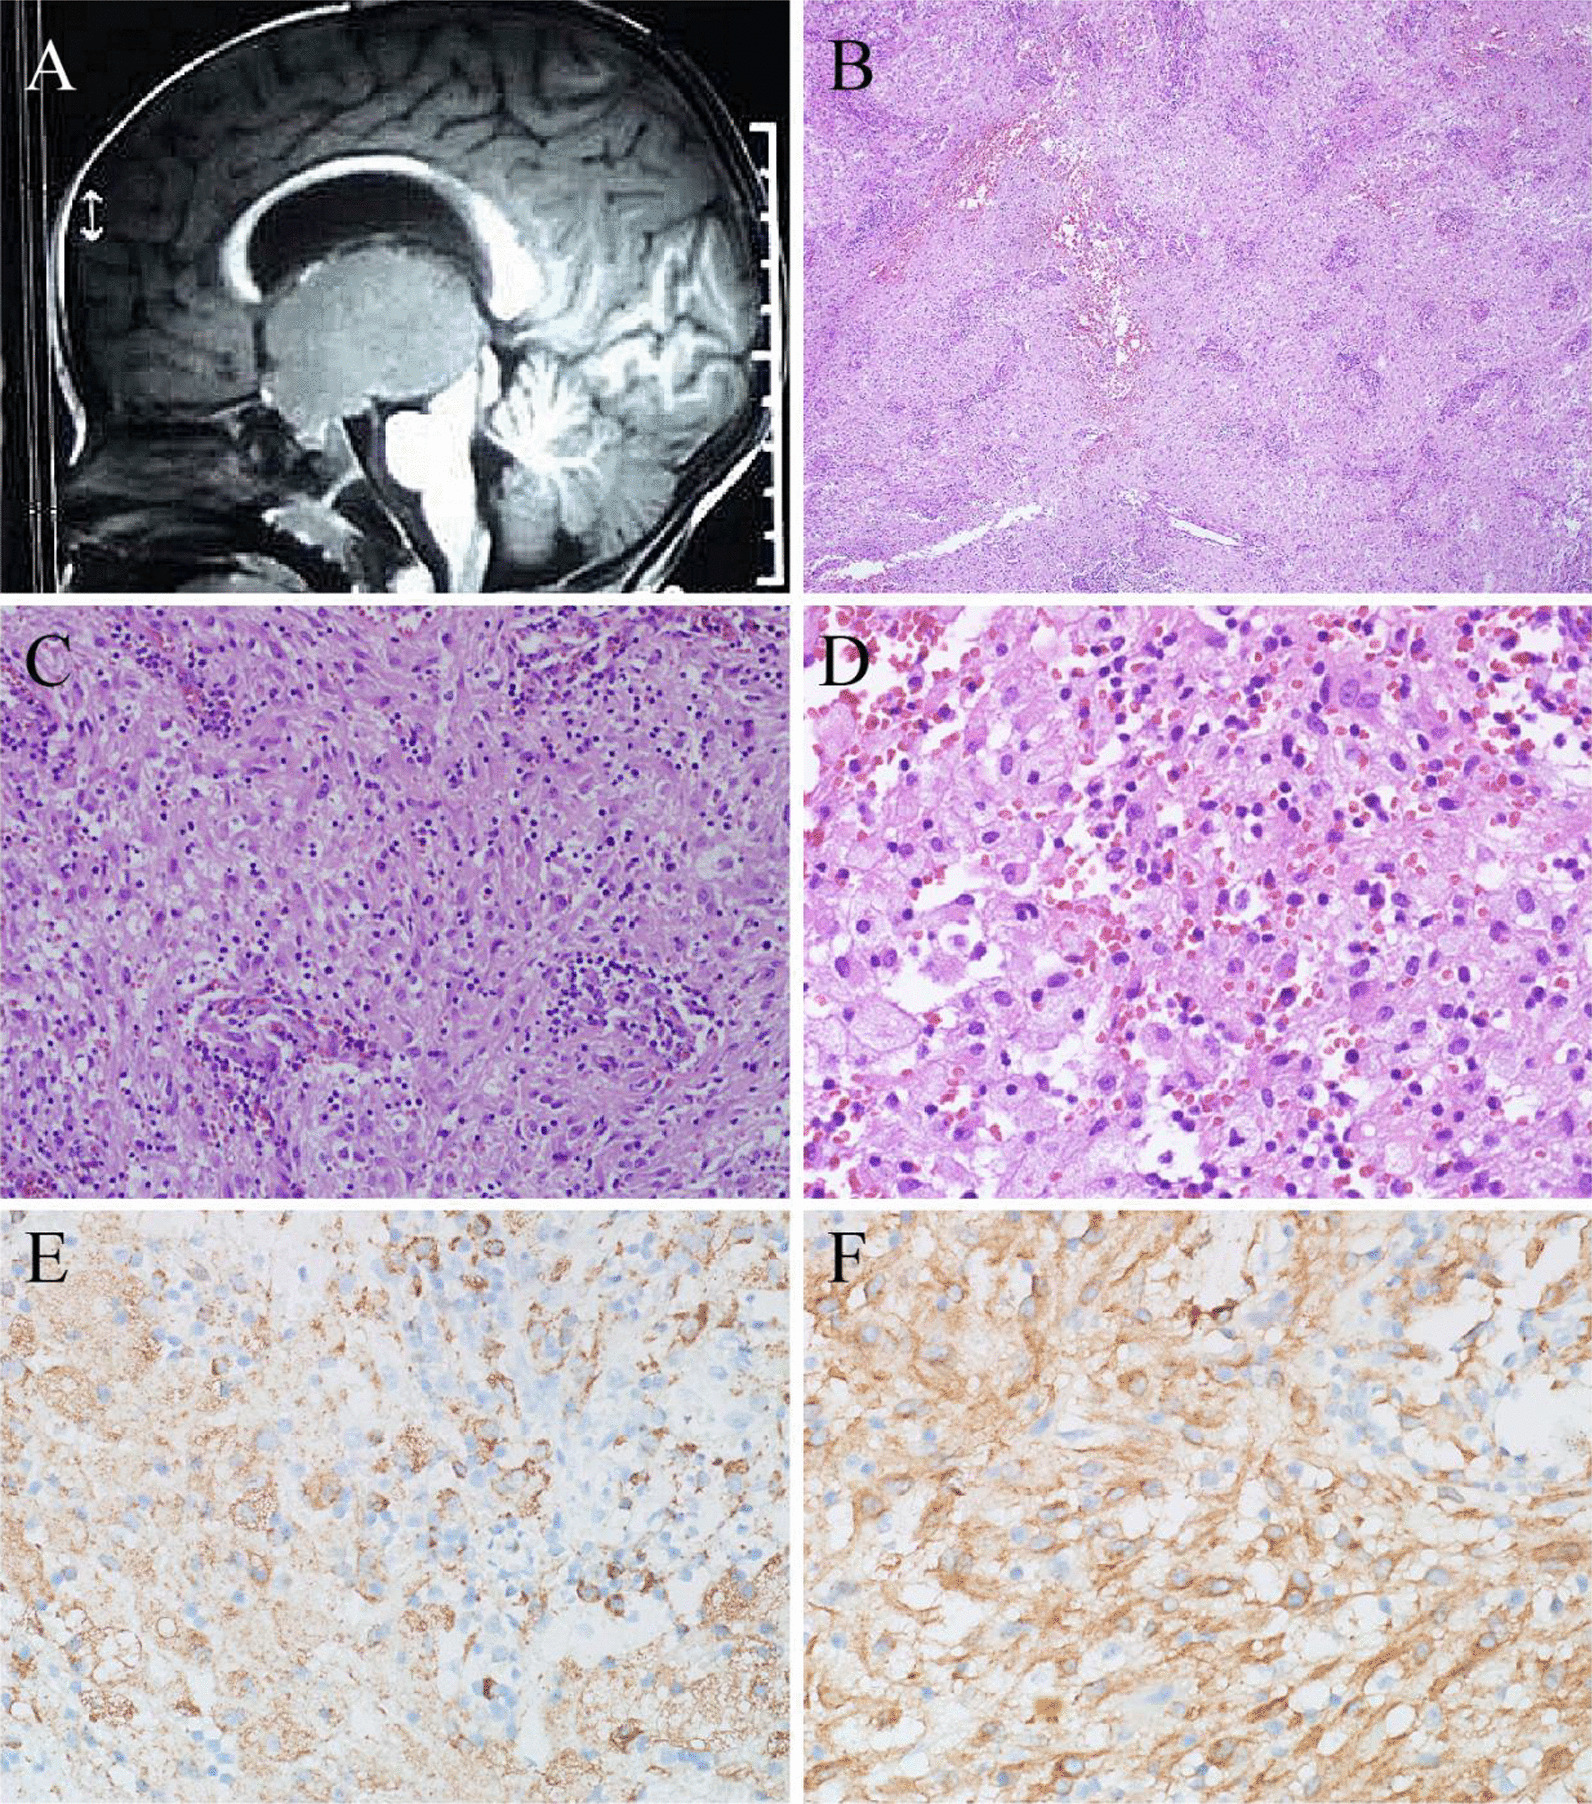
Radiological and pathological features of case 4. A The brain MRI showed a large mass (5.1 × 3.6 cm in size) in the third ventricle. B The lesion was diffusely distributed. C The histiocytes were epithelioid with abundant cytoplasm, accompanied by some lymphocytes. D Many foamy cells could be seen. E The histiocytes were positive for CD68. F The histiocytes were positive for ALK1. (Original magnification × 40 [B]; × 200 [C, E, F]; × 400 [D])
